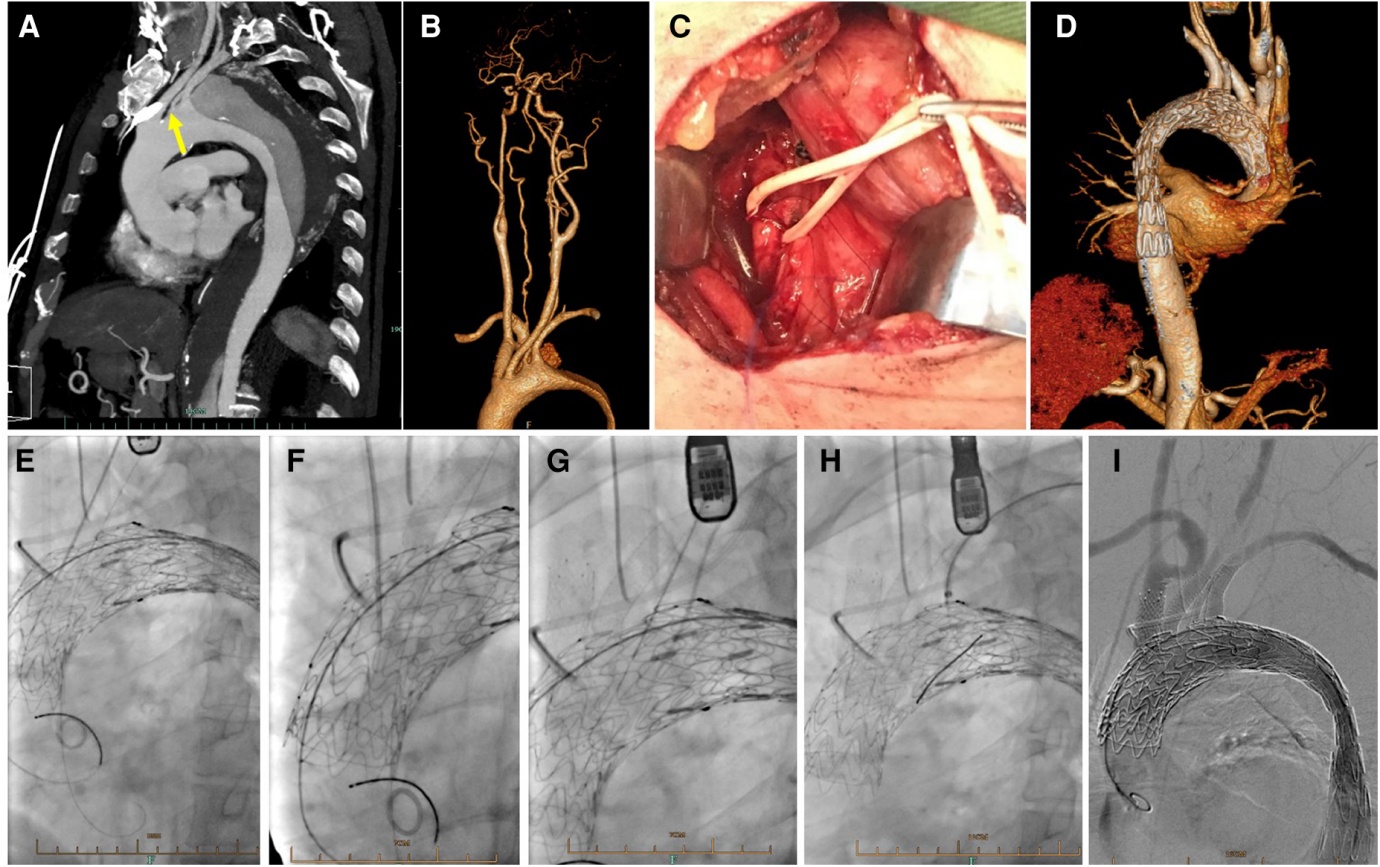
In situ* fenestration for isolated left vertebral artery. (A) Preoperative CTA showed a type B aortic dissection with ILVA (yellow arrow); (B) The cervicle contrast-enhanced CTA demonstrated dominant ILVA and incomplete circle of Willis; (C) During procedure, the ILVA was exposed with a supraclavicular incision; (D) Follow up CTA showed well exclusion of aortic dissection and patent supra aortic branches; Needle-assisted *in situ* fenestration followed by bridging stents placement for reconstruction of LCCA (E), BCT (F), ILVA (G) and LSA (H) after deployment of stent graft; (I) Complete aortography demonstrated excluded aortic dissection and patent branch vessels.
